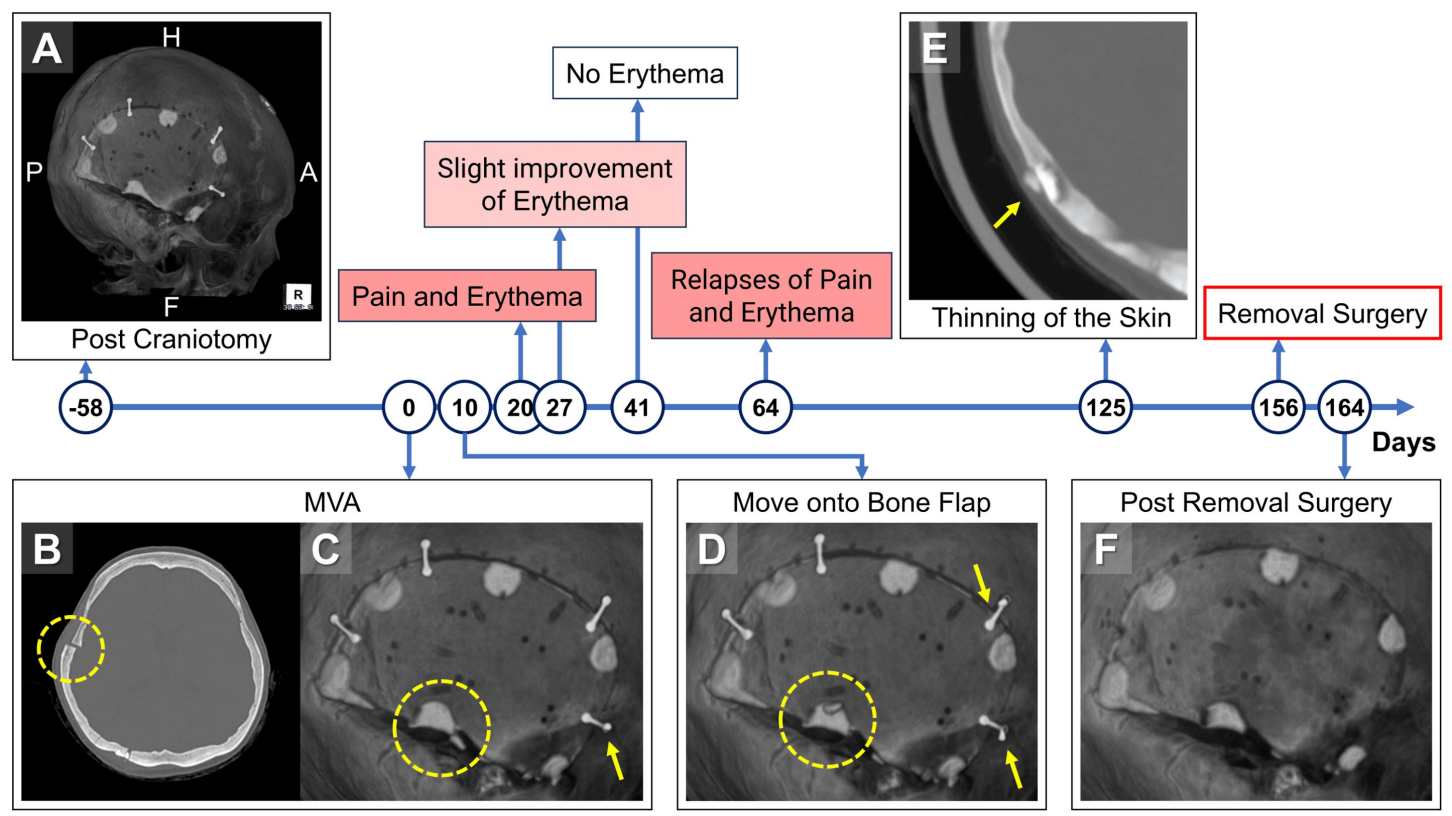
Comprehensive clinical and radiological course of this case (A) The bone flap was filled with calcium phosphate bone paste (CPBP) after resection of the cerebral arteriovenous malformation. (B, C) Following admission of the patient after a traffic accident, dislodgement of the titanium plate and screws, migration of the bone flap, and micro-fragmentation of CPBP filled with the right temporal base were apparent (circle). (D) The micro-fragments of CPBP moved onto the bone flap 10 days after admission (circle and arrows). (E) Thinning of the skin could be observed above the micro-fragmentation of CPBP four months after the accident. (F) Postoperative computed tomography after removal of micro-fragments of CPBP and the plates. MVA: motor vehicle accident
